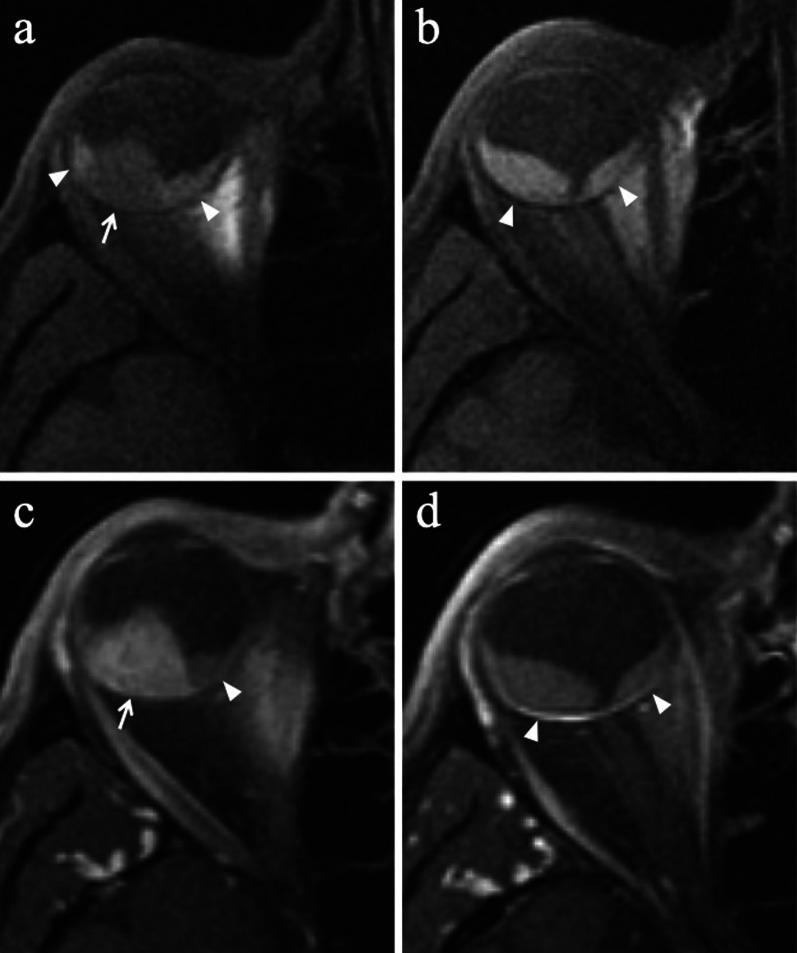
A 54-year-old man with a poorly pigmented choroidal melanoma and hemorrhagic retinal detachment of the right eye. (a, b) Axial fat-suppressed T1-weighted images demonstrate a dome-shaped intraocular mass along the postero-lateral aspect of globe (white arrow in a), displaying intermediate signal intensity because of low melanin content. A hemorrhagic retinal detachment, exhibiting the typical biconvex lentiform shape, is appreciable along the posterior aspect of globe (white arrowheads in b) and on both sides of the lesion (white arrowheads in a); the retinal detachment shows high signal intensity because of subacute hemorrhagic content. On (c, d) axial contrast-enhanced fat-suppressed T1-weighted images the choroidal melanoma exhibits vivid enhancement (white arrow in c), whereas the hemorrhagic retinal detachment does not enhance (white arrowheads)
